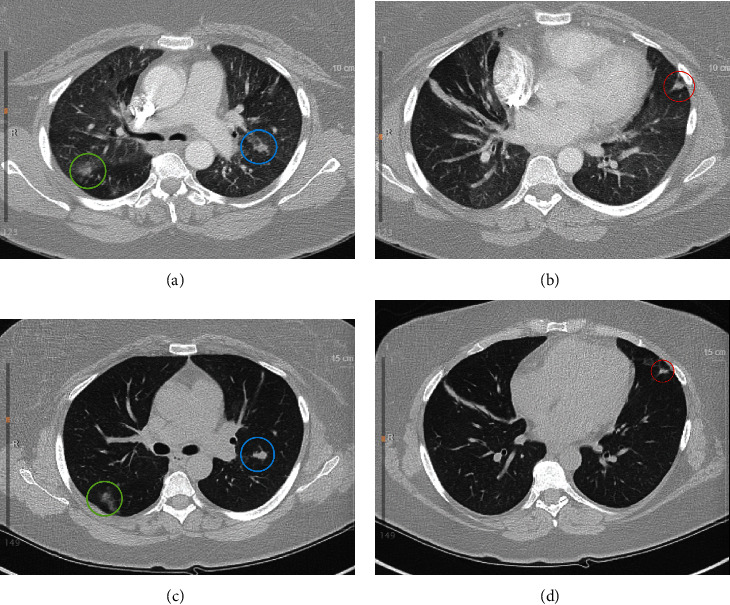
CT chest images from the initial and subsequent hospital presentations. (a) and (b) show CT scan without contrast performed during the patient's initial presentations demonstrating pulmonary nodules in the left and right upper lobes with blue and green circles, respectively, and a left pulmonary nodule at the base of the left lung with red circle. (c) and (d) show CT scan without contrast performed one month after the patient's initial consultation demonstrating similar pulmonary nodules to her preliminary visit with nodules identified by the same color circle as in (a) and (b).
